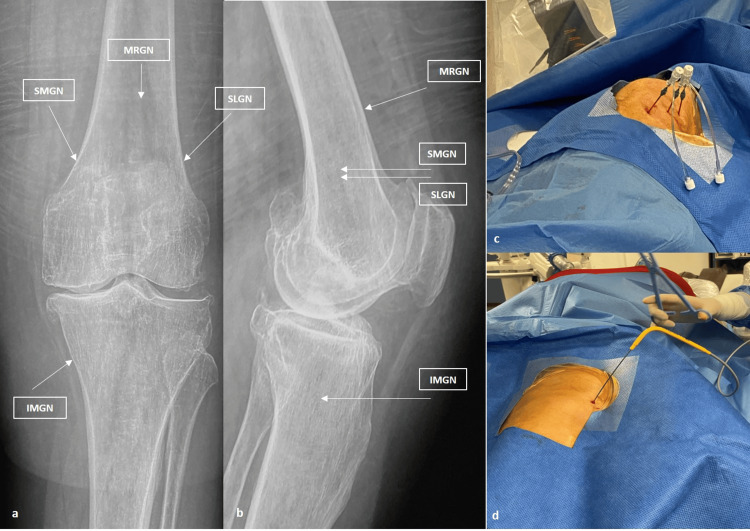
Surgical technique of ablation and targeting points. (a) Anteroposterior and (b) lateral X-rays of the knee indicating the target points of the genicular nerves; (c) intraoperative photo of the CRFA and (b) CRYO application in respect. SLGN: superior lateral genicular nerve, SMGN: superior medial genicular nerve, IMGN: inferior medial genicular nerve MRGN: medial (retinacular) genicular branch from vastus intermedius.
